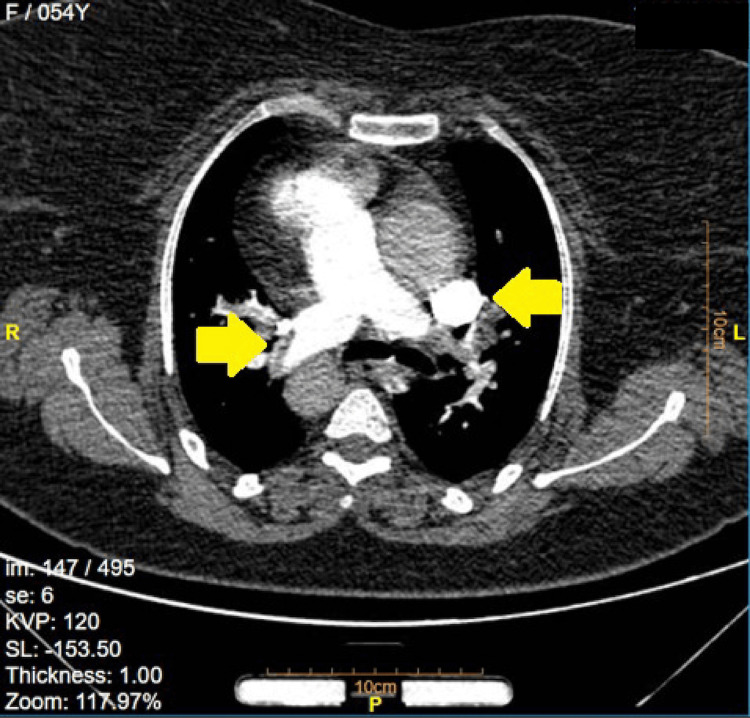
CTPA confirming massive pulmonary thromboembolism Computed tomography pulmonary angiography (CTPA) depicting filling defects (yellow arrows) indicating extensive thromboembolic burden in the right and left pulmonary arteries, consistent with massive pulmonary thromboembolism.
